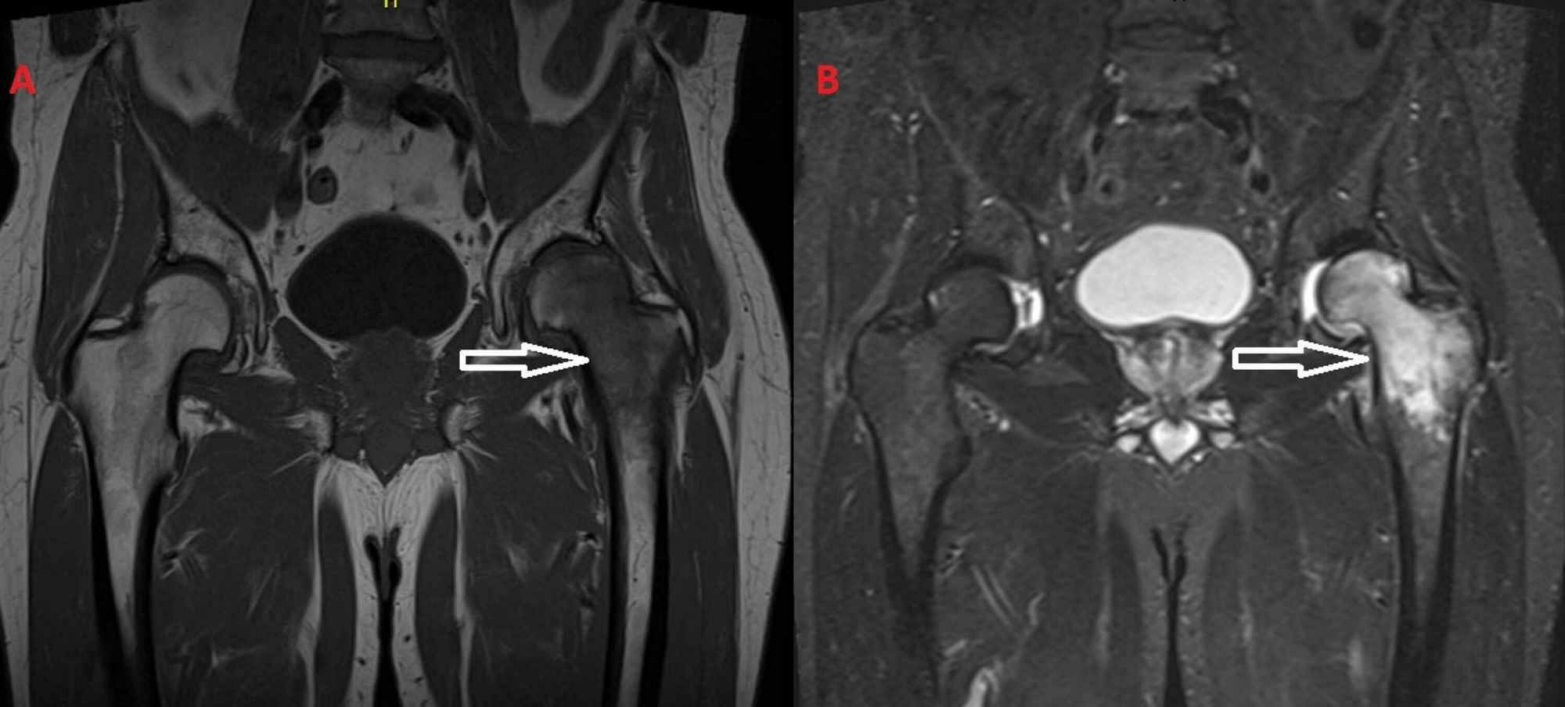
(A) T1-weighted images and (B) T2-weighted images from the MRI of the patient. The diffuse bone marrow edema of the left femoral head and the transtrochanteric region is apparent.
